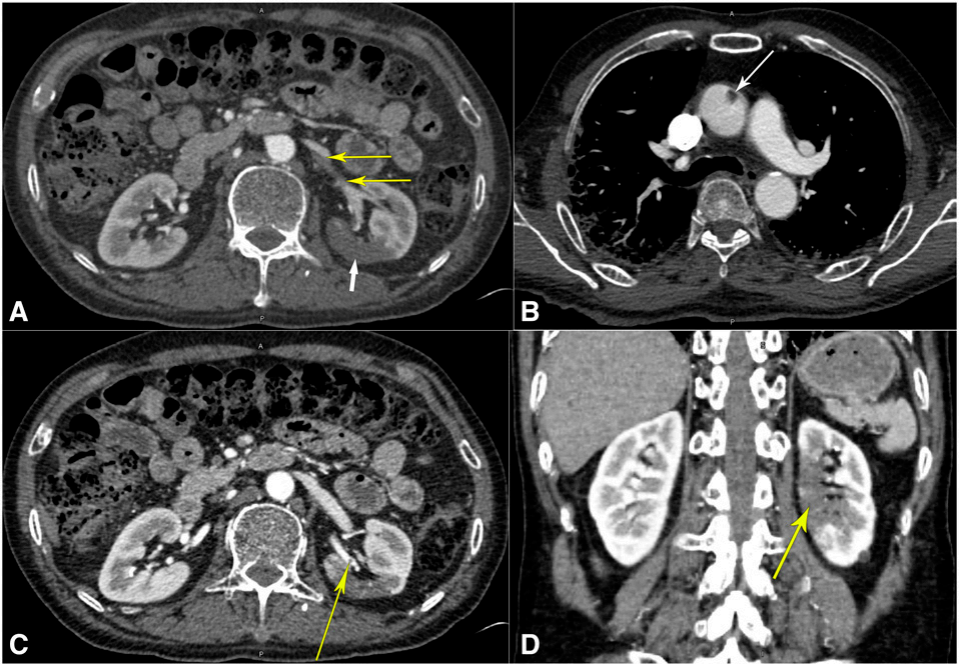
Computed tomogram with angiography. (A) Computed tomography angiogram of the abdomen showing left superior renal artery thrombi (thin yellow arrows) with infarcts in the posterior mid-pole of the left kidney (thick white arrow). (B) Computed tomography angiogram of the thorax showing the ascending aortic thrombus (arrow). (C) Computed tomography angiogram of the abdomen showing another view of the left superior renal artery thrombus (yellow arrow). (D) Computed tomography angiogram of the abdomen in the coronal view revealing the extent of the left renal infarction (yellow arrow). This figure appears in color at www.ajtmh.org.
